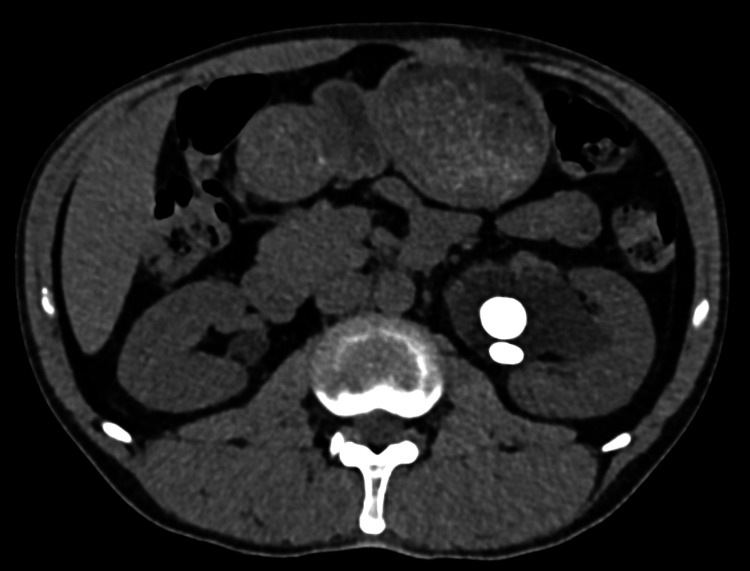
Computed tomography of abdomen showing calcification in left renal pelvis.
